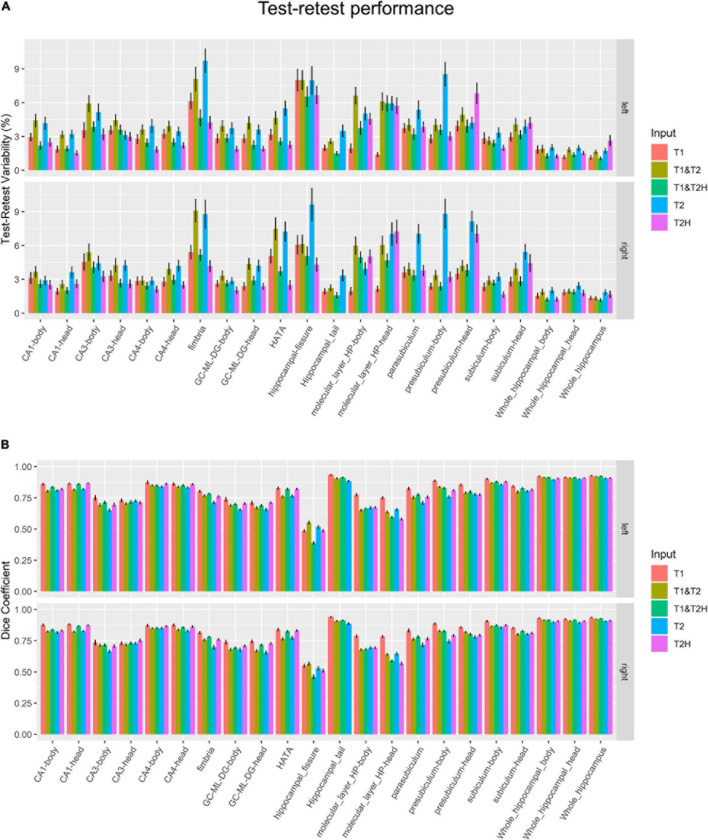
Longitudinal test-retest performance measurements. (A) Test-retest variability in percent and (B) dice coefficient metrics for each subfield and processing mode. T2H was excluded for the hippocampal tail, as not the entire structure was covered due to the limited size of the field of view. Error bars indicate standard error of the mean. T2, T2-weighted standard resolution; T2H, T2-weighted high resolution; GC-ML-DG, granule cell and molecular layer of the dentate gyrus; HATA, Hippocampus-amygdala-transition-area.
